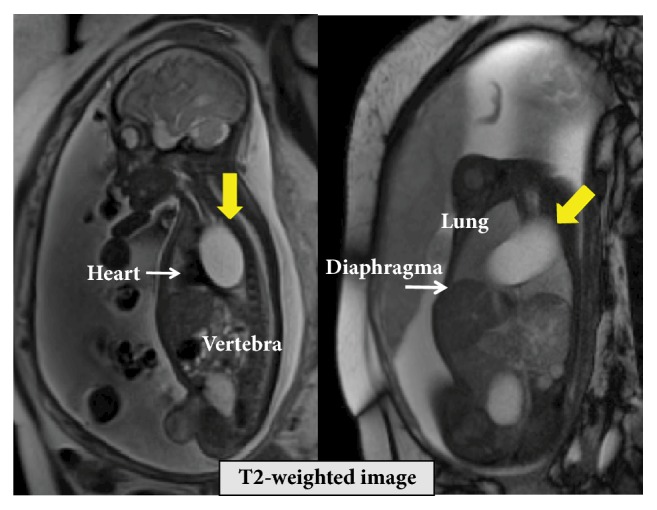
T2-weighted magnetic resonance imaging shows fetal cystic lesion located in the posterior mediastinum without communication to surrounding organs.
